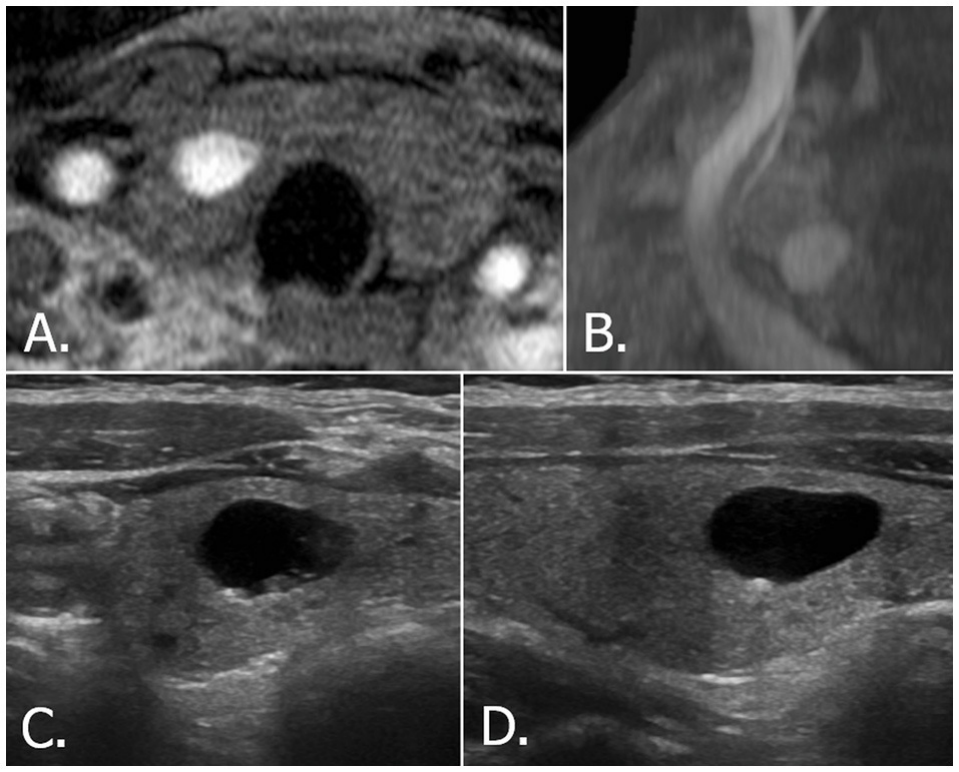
68-year-old female with right thyroid nodule. Source (A) and MIP (B) images of TOF-MR angiography show a totally hyperintense nodule in the right thyroid gland. Transverse (C) and longitudinal (D) US images of right thyroid reveals a smooth, oval, pure cystic nodule with comet tail artifact, which is classified as benign nodule (K-TIRADS category 2, ACR-TIRADS category 1 and EU-TIRADS category 2). MIP, maximum intensity projection; TOF-MR, time-of-flight magnetic resonance; US, ultrasound; K-TIRADS, Korean Thyroid Imaging Reporting and Data System; ACR-TIRADS, ACR Thyroid Imaging Reporting and Data System; EU-TIRADS, European Thyroid Imaging Reporting and Data System.
